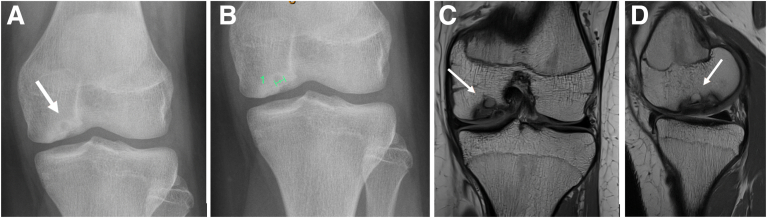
(A) Plain anteroposterior radiograph showing irregular distal medial femoral joint line, suspicious of osteochondritis dissecans (OCD), with the area of concern demarcated. These appearances warrant further investigation with magnetic resonance imaging (MRI). (B) Plain lateral radiograph taken preoperatively of a symptomatic patient showing a demarcated OCD lesion. (C, D) T1-weighted coronal and sagittal MRI sequence showing a medial femoral condyle OCD unstable lesion prior to any surgical intervention.
